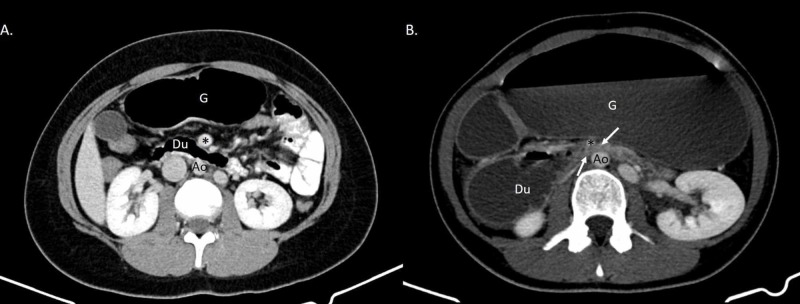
IV contrast-enhanced axial computed tomography (CT) of the abdomen and pelvis consistent with superior mesenteric artery syndrome, with eight-month comparison. Ao: aorta, asterisk: superior mesenteric artery (SMA), Du: duodenum, G: stomach. A. This figure demonstrates normal bowel eight months prior to the patient's acute presentation. It was performed due to right lower quadrant abdominal pain. B. This figure reveals dilated stomach and proximal duodenum with air fluid levels. There is an abrupt collapse of the third portion of the duodenum as it crosses posterior to the SMA close to midline. The arrows denote the transition point between dilated and collapsed small bowel.
